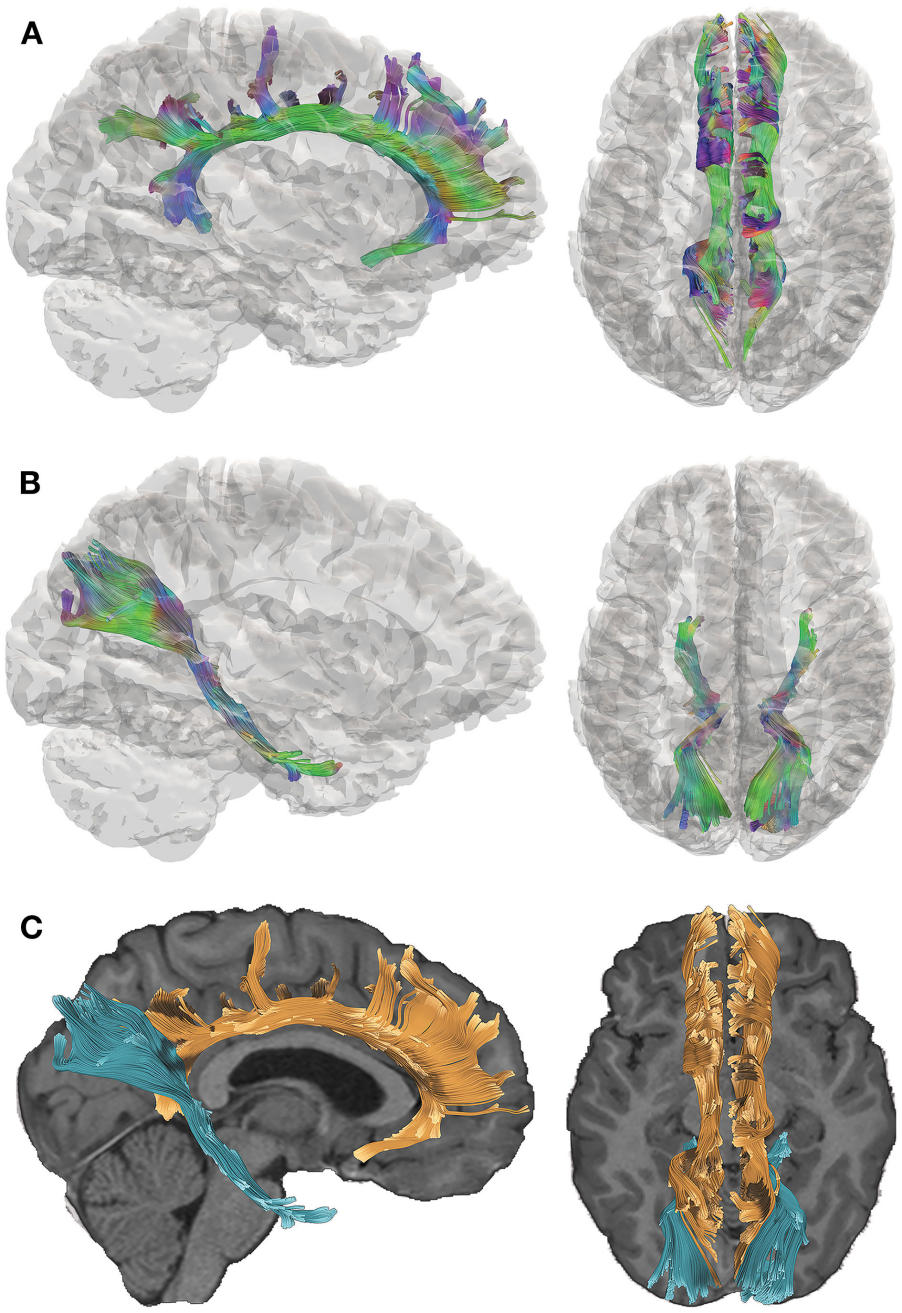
Tractography of the cingulum bundle (CB) and perforant pathway (PP). Directional color maps of the right CB (A) and PP (B) are shown on the sagittal (left) and axial (right) planes, overlaid by a 3D cortical isosurface. The colors represent the 3D fiber rendering direction; red, left-to-right (along the *x*-axis); green, anterior-to-posterior (along the *y*-axis); and blue, superior-to-inferior (along the *z*-axis). The bottom panel (C) demonstrates the spatial relationship of the CB (orange) and PP (blue), which are overlaid on the subject's T1-weighted image. The right side of the brain is depicted in the left side of the screen in the axial views.
